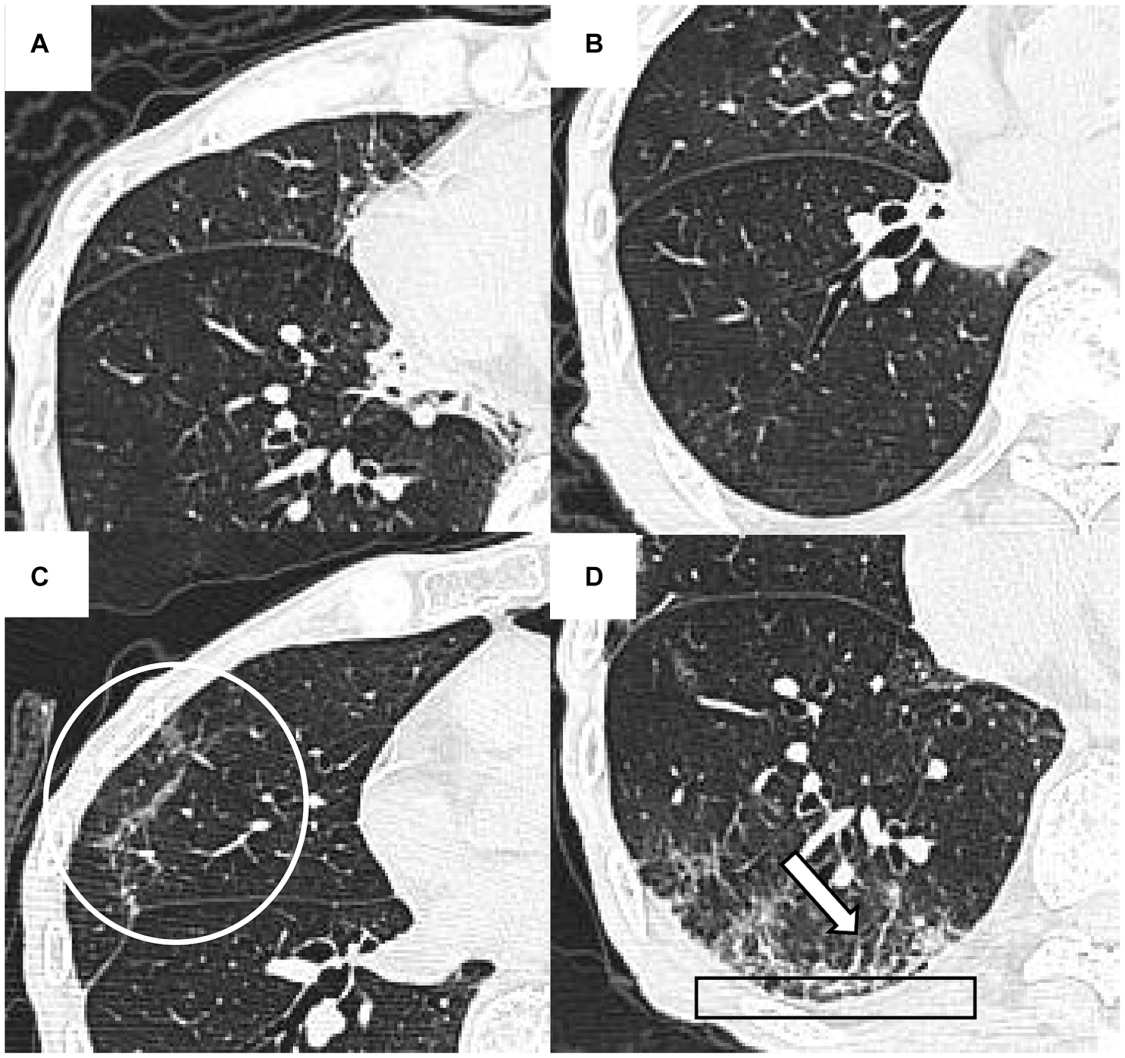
The initial and 48-day follow-up CT findings of a 93-year-old man with COVID-19 pneumonia. The initial CT scans (A,B) did not reveal any signs of fibrotic interstitial lung abnormalities (ILAs). However, the follow-up CT (C,D) showed fibrotic ILAs at the identical anatomical level. These changes include architectural distortion (indicated by a circle in C), reticulation (outlined by a rectangle in D), and traction bronchiectasis and/or bronchiolectasis (marked by an arrow in D).
